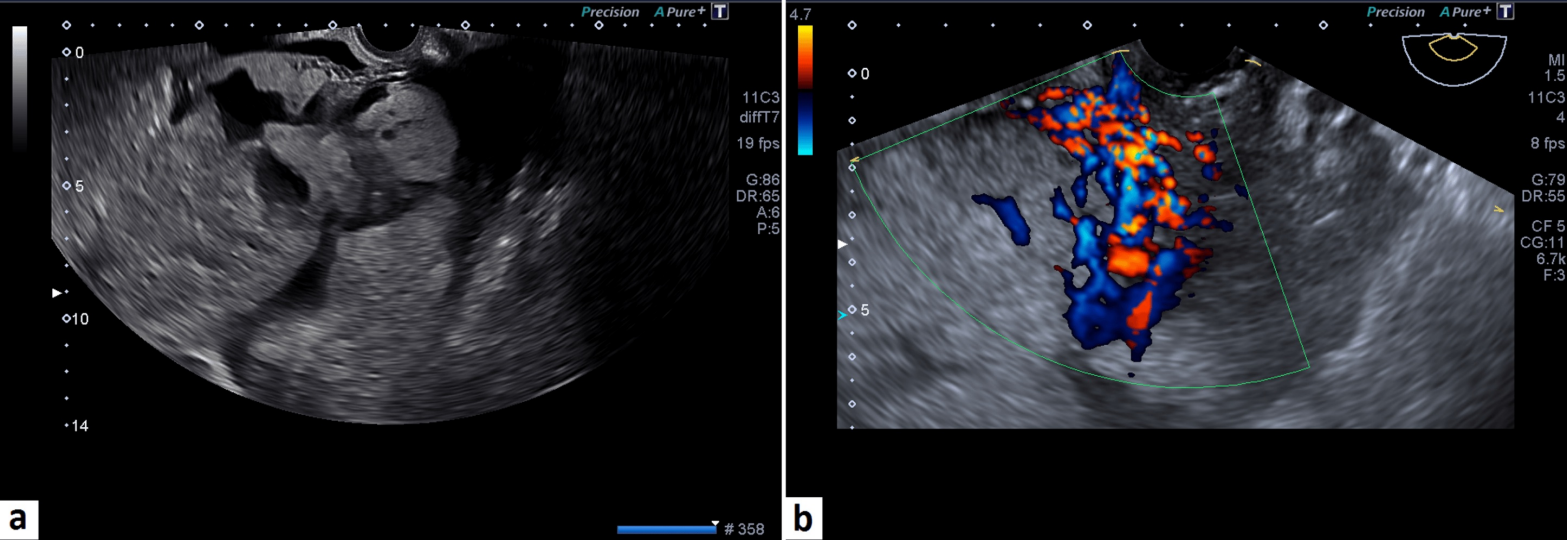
(a) Ultrasound image of PAS showing multiple placental lacunae and interruption of uterovesical interface. (b) Color Doppler image of PAS showing subplacental hypervascularity (in the inferior part of lower uterine segment). PAS, placenta accreta spectrum
